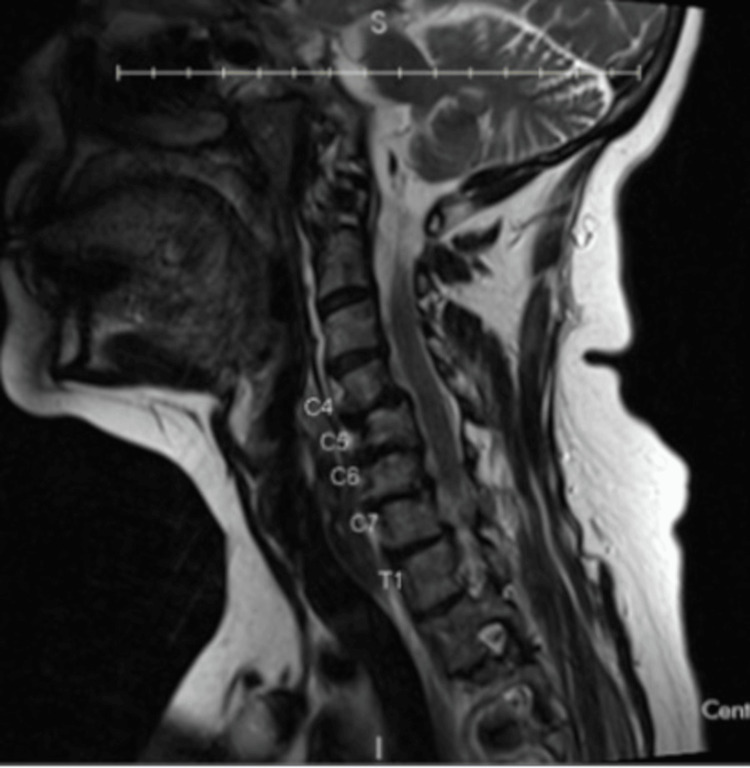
Initial Mid-sagittal T2-Weighted MRI MRI obtained three years prior demonstrating mild spinal canal stenosis and C4-5 spondylolisthesis without facet dislocation.
